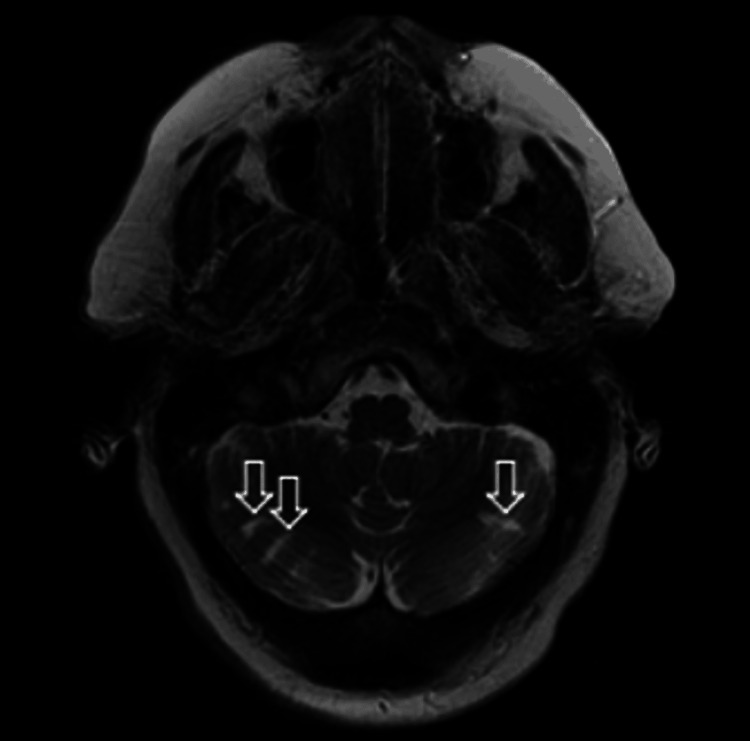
Apparent diffusion coefficient MRI of the brain showing increased signal intensity in the cerebellum bilaterally due to chronic infarcts (open arrows).
